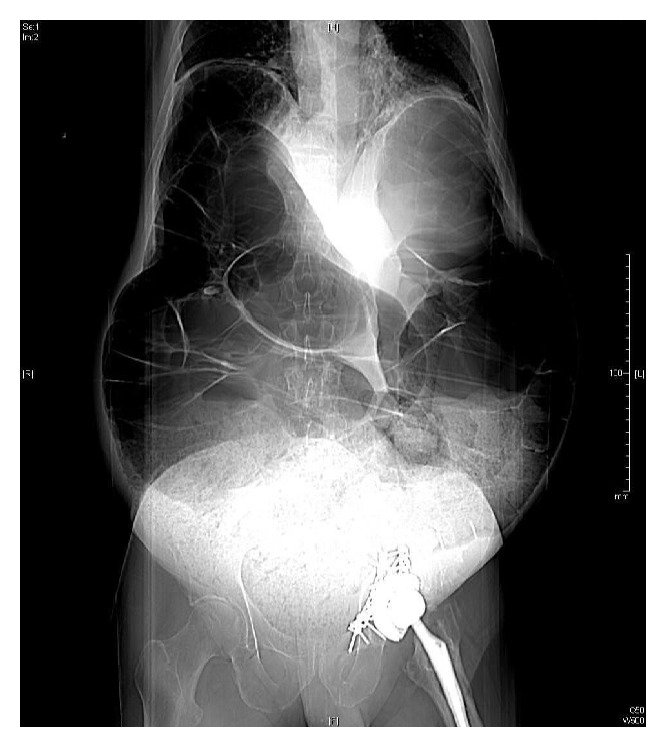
CT scout film showing dilated loops of bowel.
